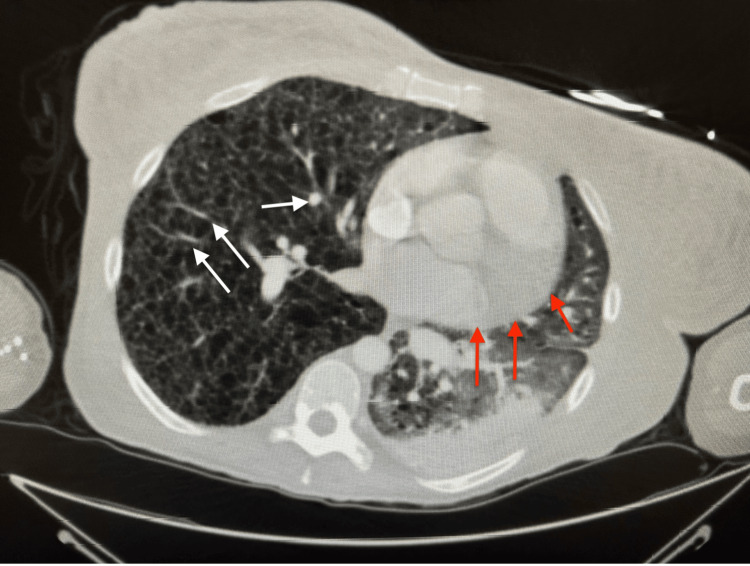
Chest CT confirms left lower lobe PNA and multiple sclerotic foci CT: Computed tomography; L: Left; PNA: Pneumonia; Red arrows: Lobar PNA; White arrows: Sclerotic foci
